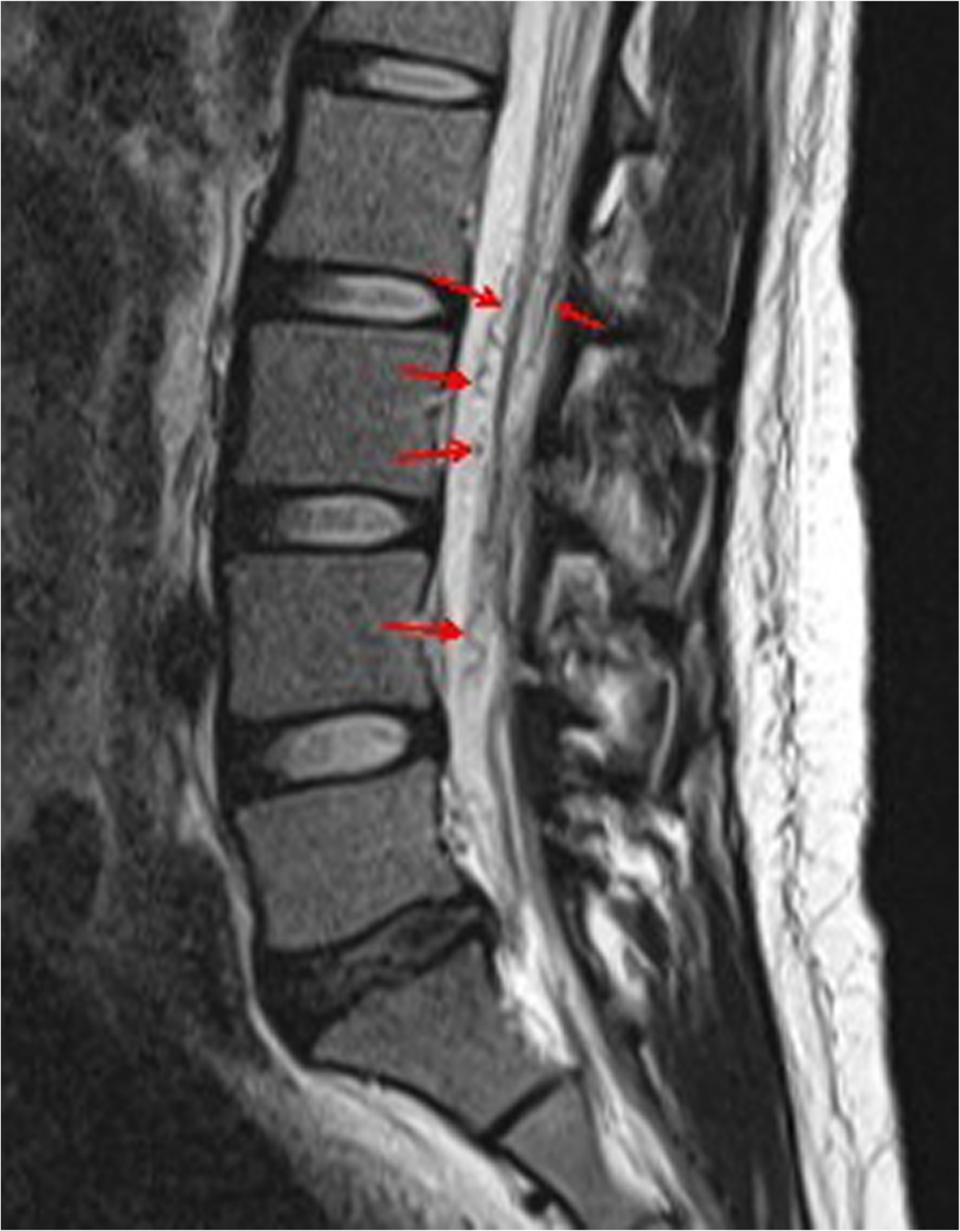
Magnetic resonance imaging (T2-weighted image) in the lumbar level at 32 weeks of gestation. Flow voids (the arrows) suggest dilated draining veins
